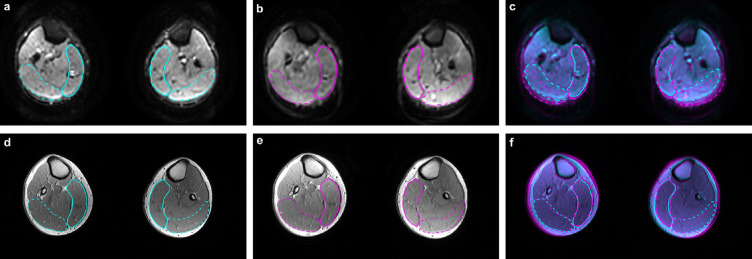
Spatial distortion in magnetic resonance images acquired off-isocentre. Axial echo-planar imaging (a-c) and 3D gradient echo (d-f) images were acquired in a bilateral setup. The gastrocnemius medialis and lateralis muscles are outlined with a solid and dashed line, respectively. Images (a) and (d) show the slice positioned at the isocentre, while in images (b) and (e) the slice was located at 14.4 cm off-isocentre. The overlays (c, f) demonstrate substantial spatial distortion [(a, d) in cyan; (b, e) in magenta]. Images were taken from a 37-year-old man (body weight and height: 76 kg, 182 cm). Image settings are listed in Table S1 (IDs 1 A, 1B, 2 A, and 2B).
